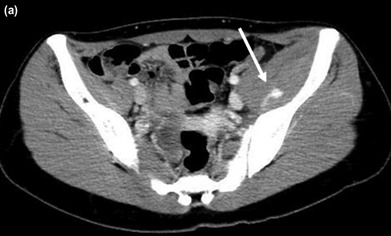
Contrast-enhanced computed tomography scan showing extravasation in the iliacus hematoma (white arrow).
